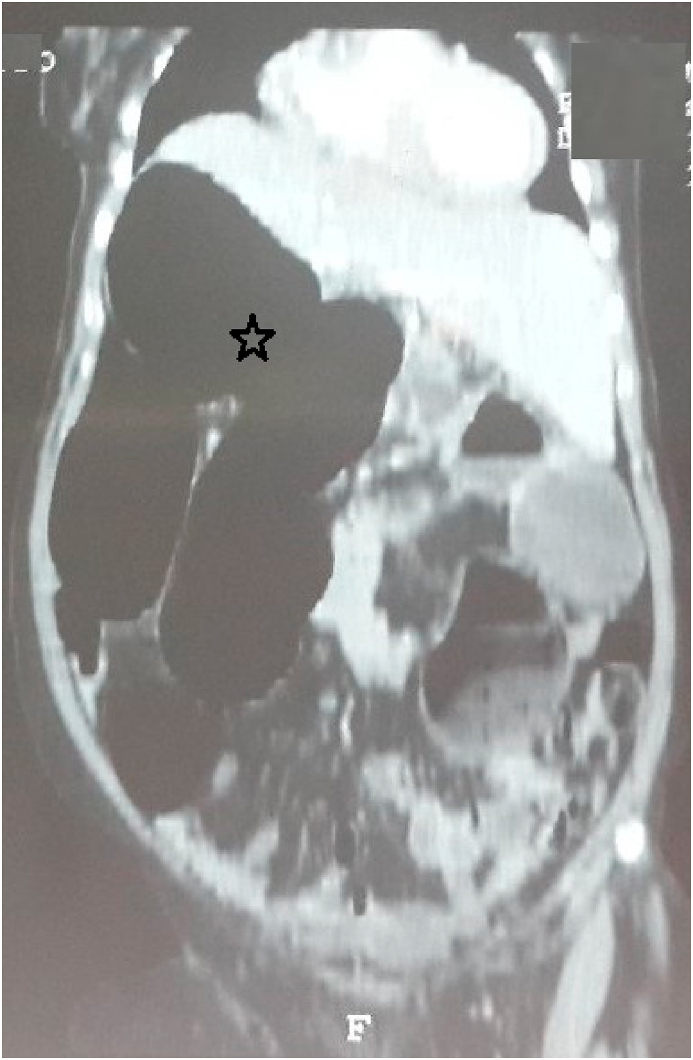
CT scan of the abdomen (coronal plane) showing a “U-shaped” loop of dilated colon (star) in the right upper abdomen.
